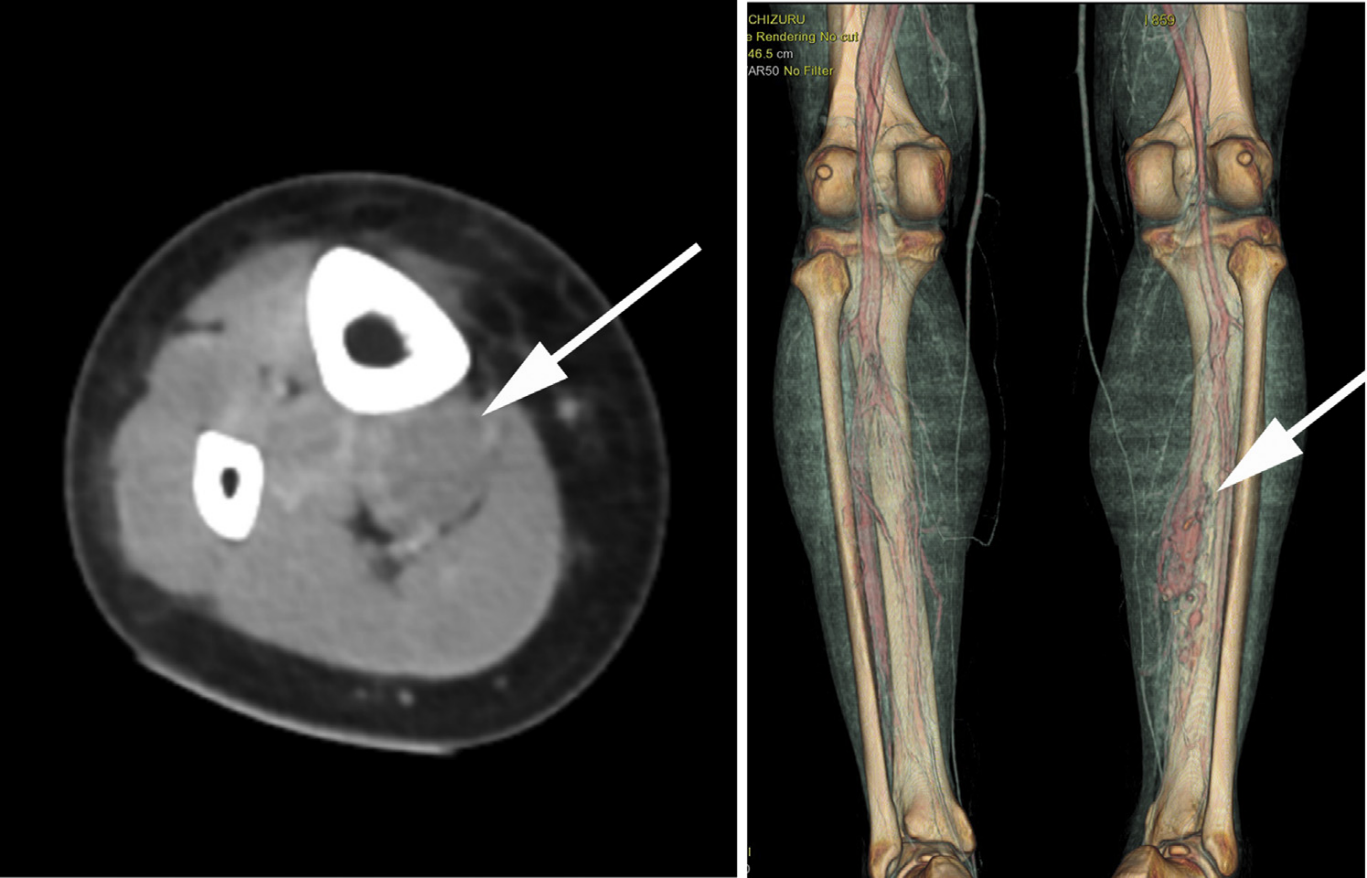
Left, Contrast-enhanced computed tomography image showing the posterior tibial vein aneurysm (*white arrow*) in the axial view. Right, Three-dimensional computed tomography image showing posterior tibial vein aneurysm (*white arrow*) and collateral venous circulation.
